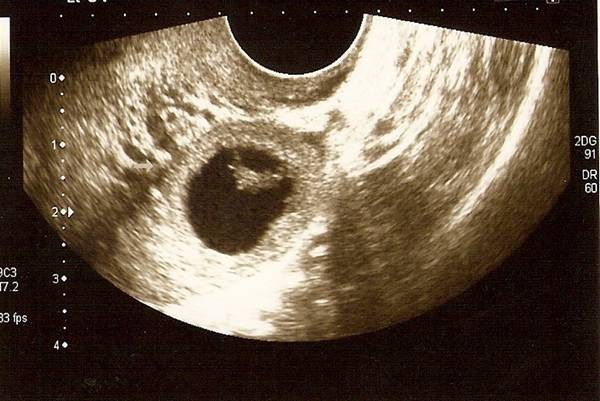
Transvaginal ultrasound showing left adnexal mass containing gestational sac with fetal pole.
